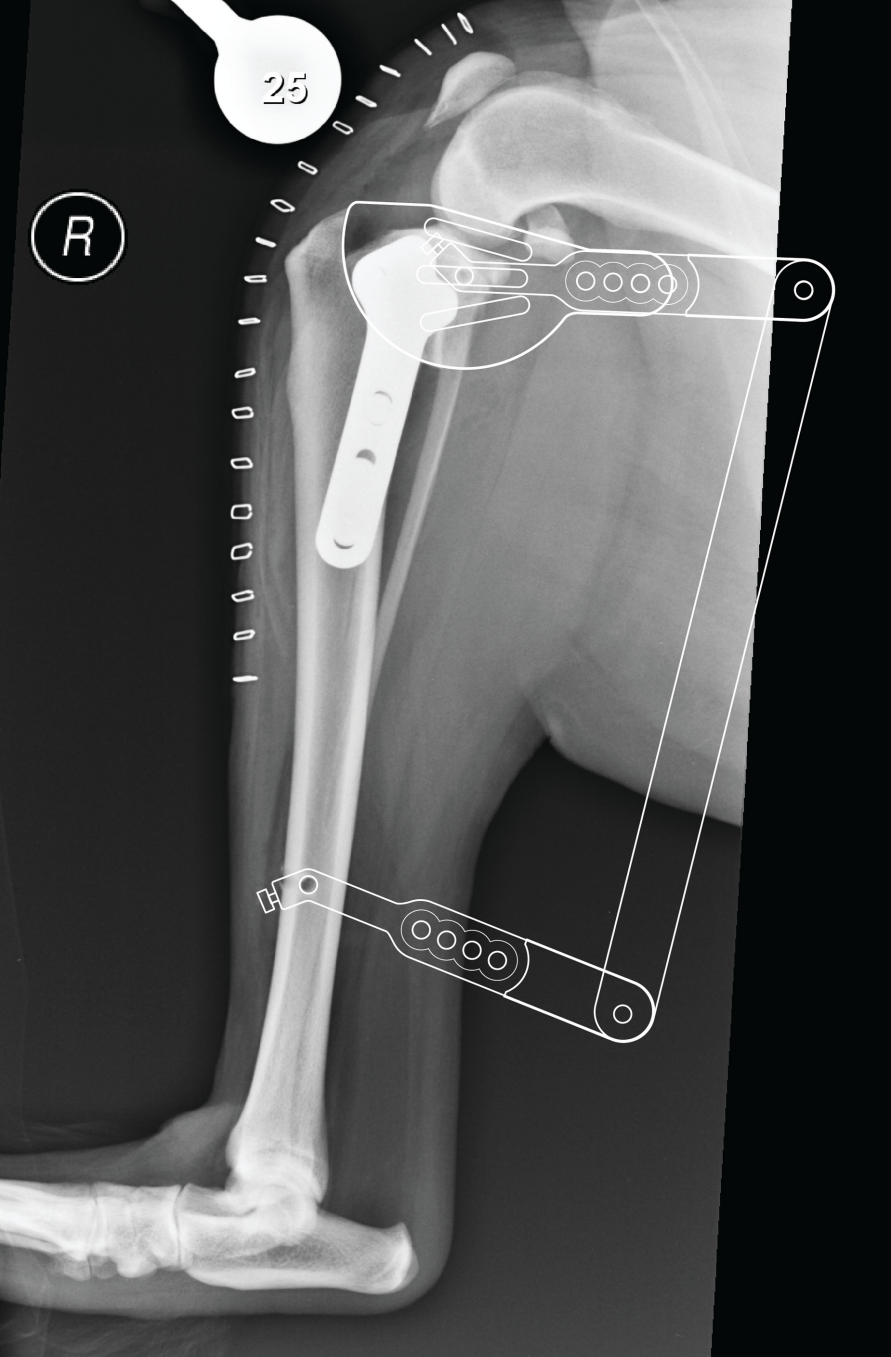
Digital representation of the method used to align the jig and guide onto a true-to-size printed postoperative lateral radiograph. The alignment jig/saw guide was aligned with the long axis of the tibia using the proximal and distal jig pin holes. The saw guide was then positioned to achieve the best fit over the osteotomy by angling the jig arms (see Fig 3). The widest offset along the radial osteotomy between the actual and saw guide osteotomy position was recorded (≤1 mm = excellent; ≤2 mm = good; ≤3 mm = satisfactory; >3 mm = poor).
